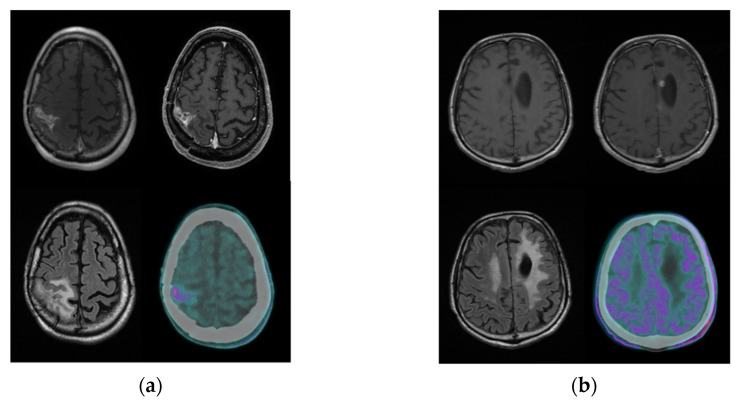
Representative examples of two patients with previous history of surgically treated grade IV HGG investigated with MET-PET scan for a suspicion of recurrence (T1-weighted, contrast-enhanced T1-weighted and FLAIR MR images and MET-PET images). In patient (a), MET-PET shows focal uptake higher than background in the right fronto-parietal region, corresponding to the site of abnormal signal intensity detected on MR and suspected for relapse; the T/B and SUVmax_TTP_ values were 3.94 and 15.58, respectively. Recurrence was confirmed by surgery 1 month after MET-PET evaluation, and the patient died 10 months later. In patient (b), the MET-PET scan did not show any area of focal uptake higher than background, especially in the area of abnormal signal intensity detected at MR on the left medial paratrigonal region and suspected for relapse; the T/B and SUVmax_TTP_ values were 1.45 and 8.64, respectively. The patient had no recurrence and was still alive at the time of the last follow-up.
